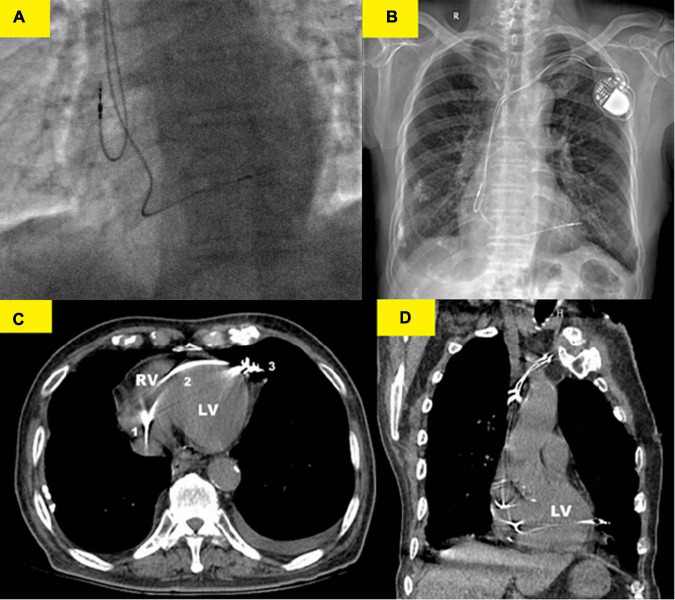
Various imaging modalities showed the lead perforation. (A) X-ray fluoroscopy image (right anterior oblique 30°) immediately after implantation showed that the RV lead was placed at the right ventricular (RV) apex. (B) Chest x-ray showed that the RV lead was beyond the left cardiac margin and clearly migrated in the left hemithorax. (C) Thoracic computed tomography (CT) scan showed the RV lead course from the right ventricle (1) to the left cardiac margin (3) in the patient and perforation of the interventricular septum (2) was well-evident. (D) Coronal CT images confirmed the RV lead migration in the left hemithorax. Thoracic CT scan should, therefore, be regarded as the gold standard for the strategical management of this complication.
